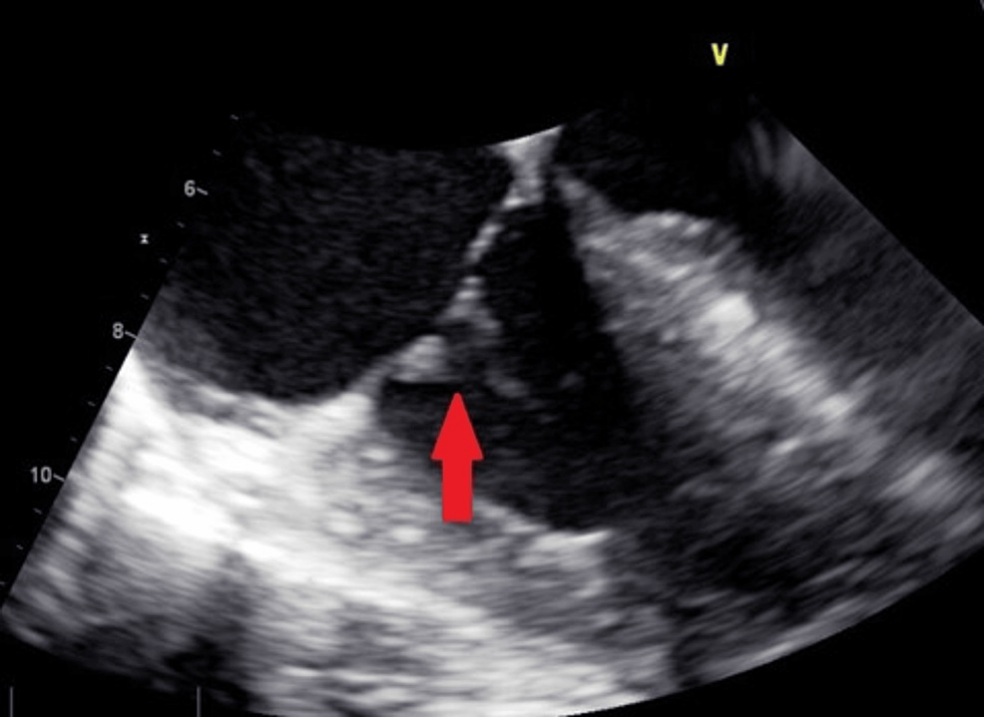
Transesophageal echocardiogram (TEE) image demonstrating a 0.9x0.6 cm vegetation attached to the septal annulus of the tricuspid valve (arrow).
